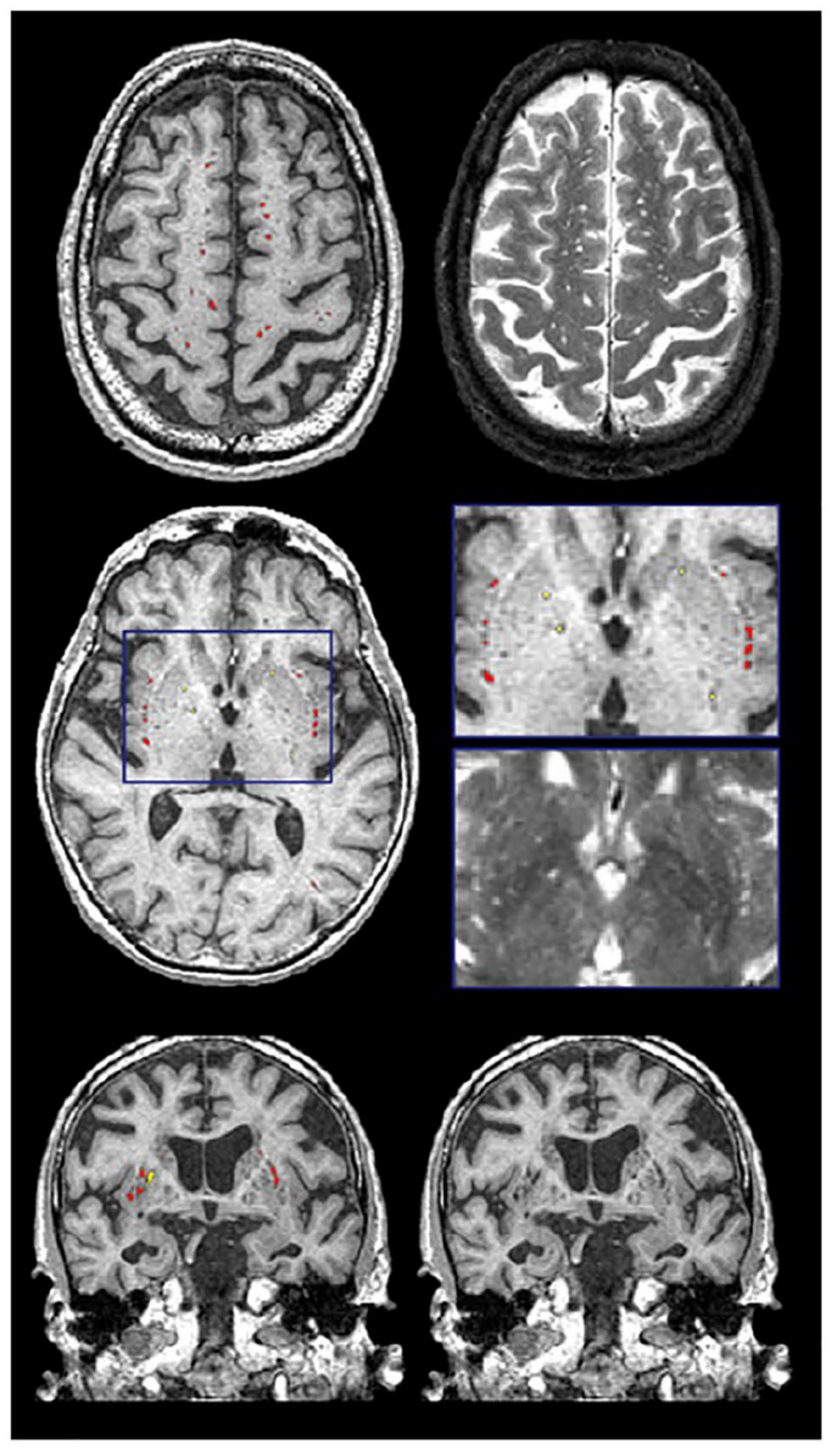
Examples of the PVS segmentation (red and yellow) over-layed onto structural MRI in axial (top 2 rows) and coronal views (bottom row).
